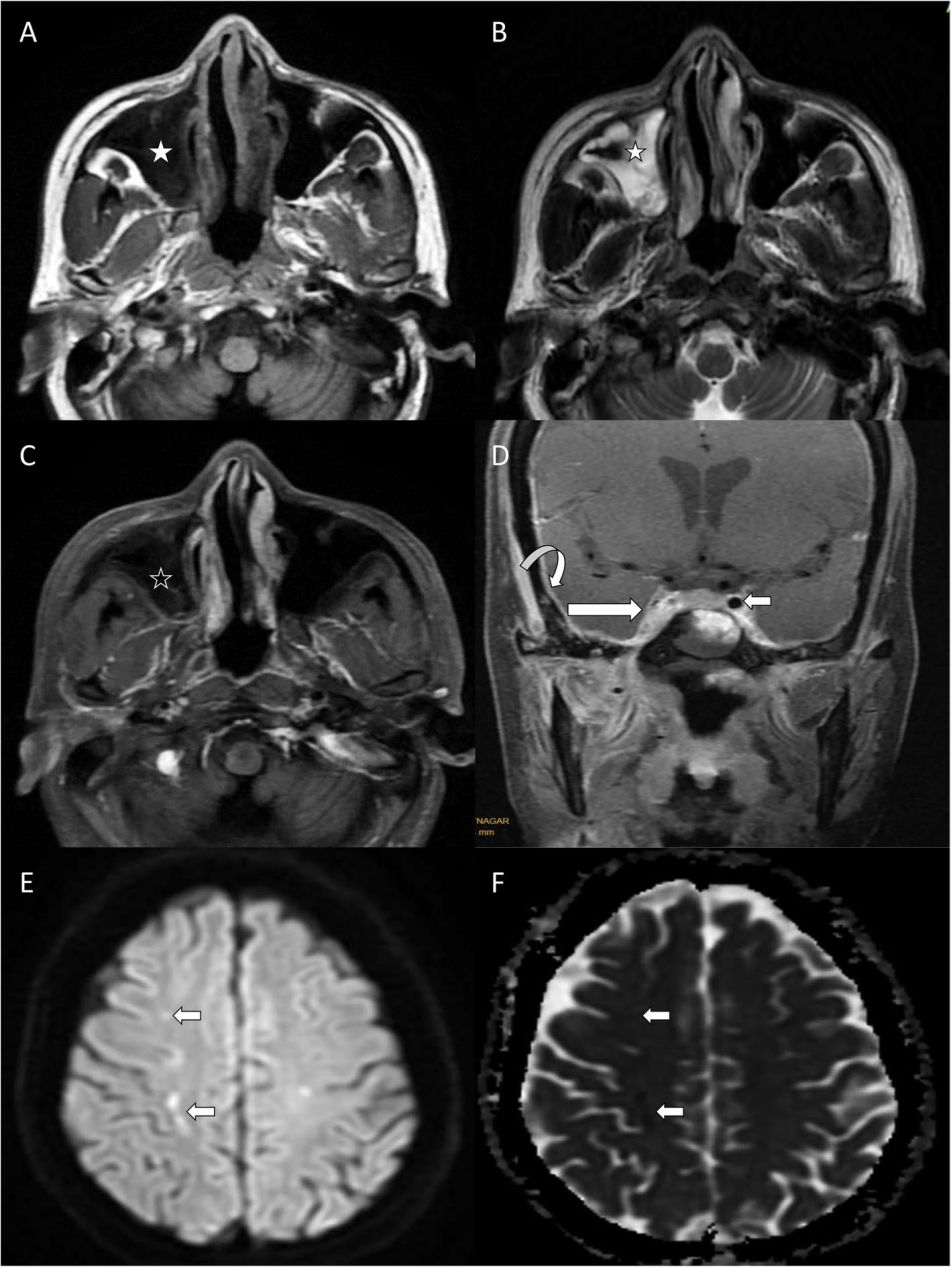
Extension of sinonasal disease into the cavernous sinus. Axial T1- and T2-weighted images (A and B) show thickening of the right maxillary sinus wall (white stars) which shows no enhancement (black star) on axial contrast-enhanced fat-saturated T1-weighted image (C). Coronal contrast-enhanced fat-saturated T1-weighted image (D) shows non-enhancing areas within the right cavernous sinus with convex outward margin (straight long arrow), suggestive of cavernous sinus thrombosis. The flow void of the cavernous part of the right internal carotid artery is not seen (straight white arrow shows normal flow void of the internal carotid artery on the left side). The meninges appear thickened and show enhancement (curved arrow) indicating meningitis. Axial diffusion-weighted (E) and apparent diffusion coefficient (F) images show foci of restricted diffusion (straight short arrows) in the right centrum semiovale in the watershed territory, suggesting cerebral infarcts
